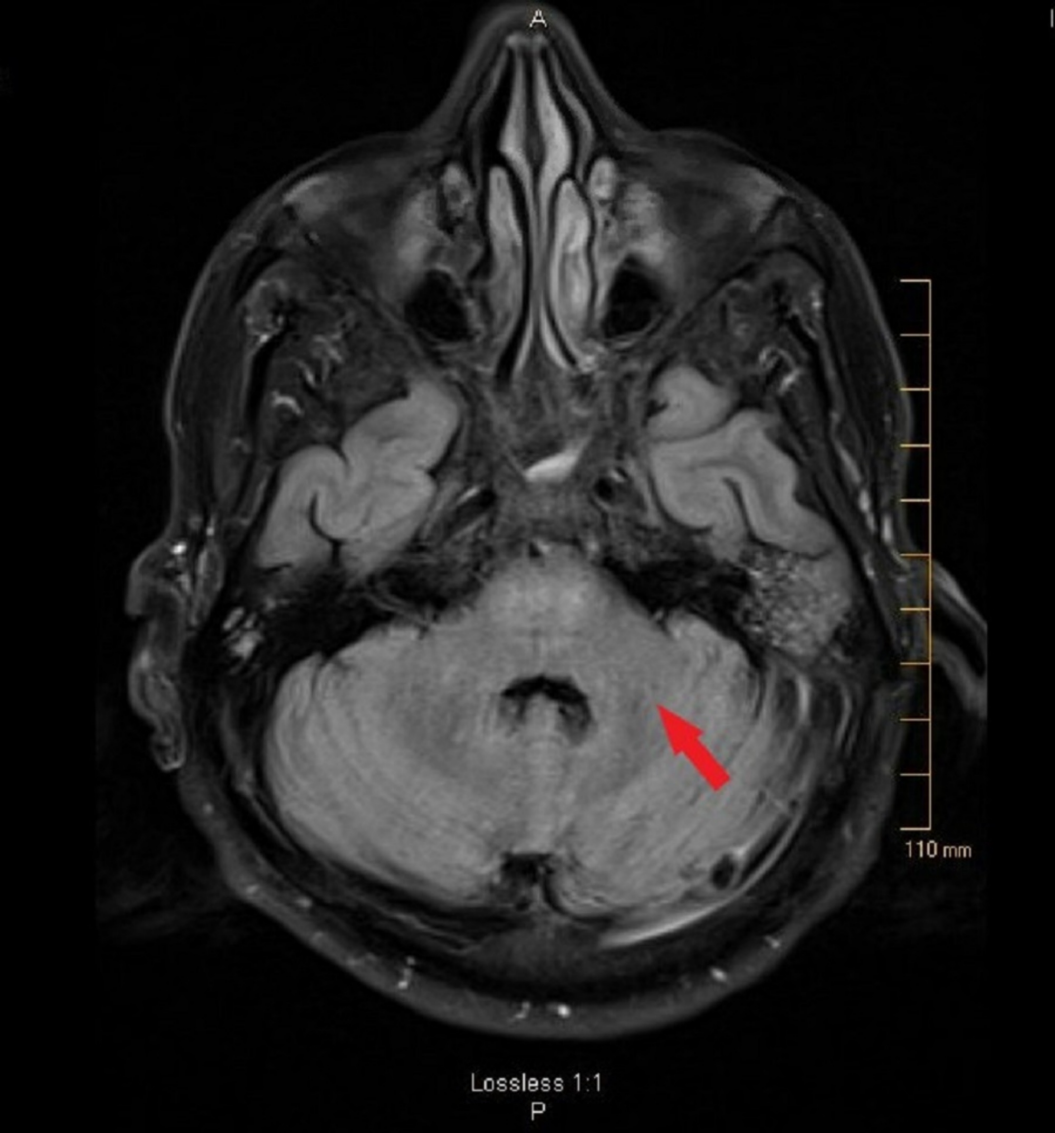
Follow-up MRI shows marked regression of lesions involving the brainstem and cerebellar peduncles on FLAIR sequence (red arrow). MRI: Magnetic resonance imaging; FLAIR: Fluid-attenuated inversion recovery.
